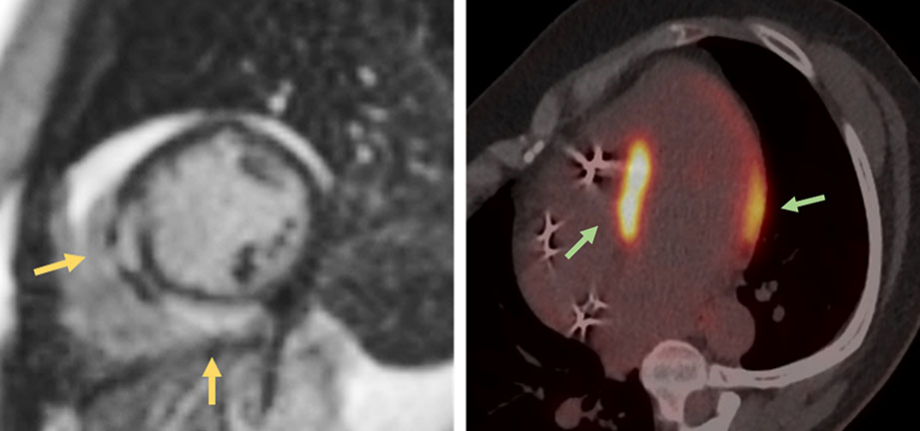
The patient’s initial diagnosis of cardiac sarcoidosis 6 years ago was supported by both cardiovascular magnetic resonance imaging (displayed on the left) and ^18^F-Fluorodeoxyglucose (^18^F-FDG) positron emission tomography scan (displayed on the right). The cardiac MRI shows typical late gadolinium enhancements in the left ventricular septum and posterior wall (indicated by yellow arrows). The ^18^F-FDG PET scan also shows abnormal activity, particularly in the basal septum and lateral wall (indicated by green arrows)
